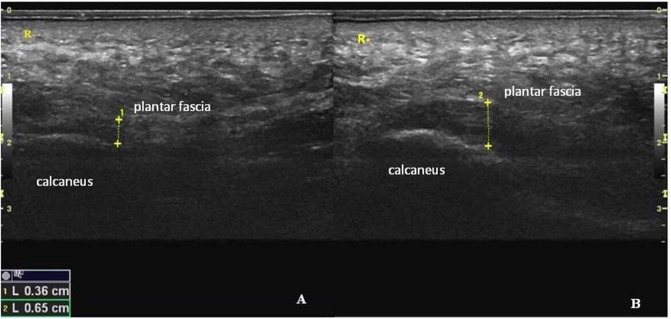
An example of a longitudinal sonogram of the plantar fascia with the corresponding thickness*. The* thickness of the plantar fascia was measured at the anterior margin of the calcaneus. (A) Thickness of the plantar fascia of a healthy subject. (B) Thickness of the plantar fascia in *patient* with PF.
